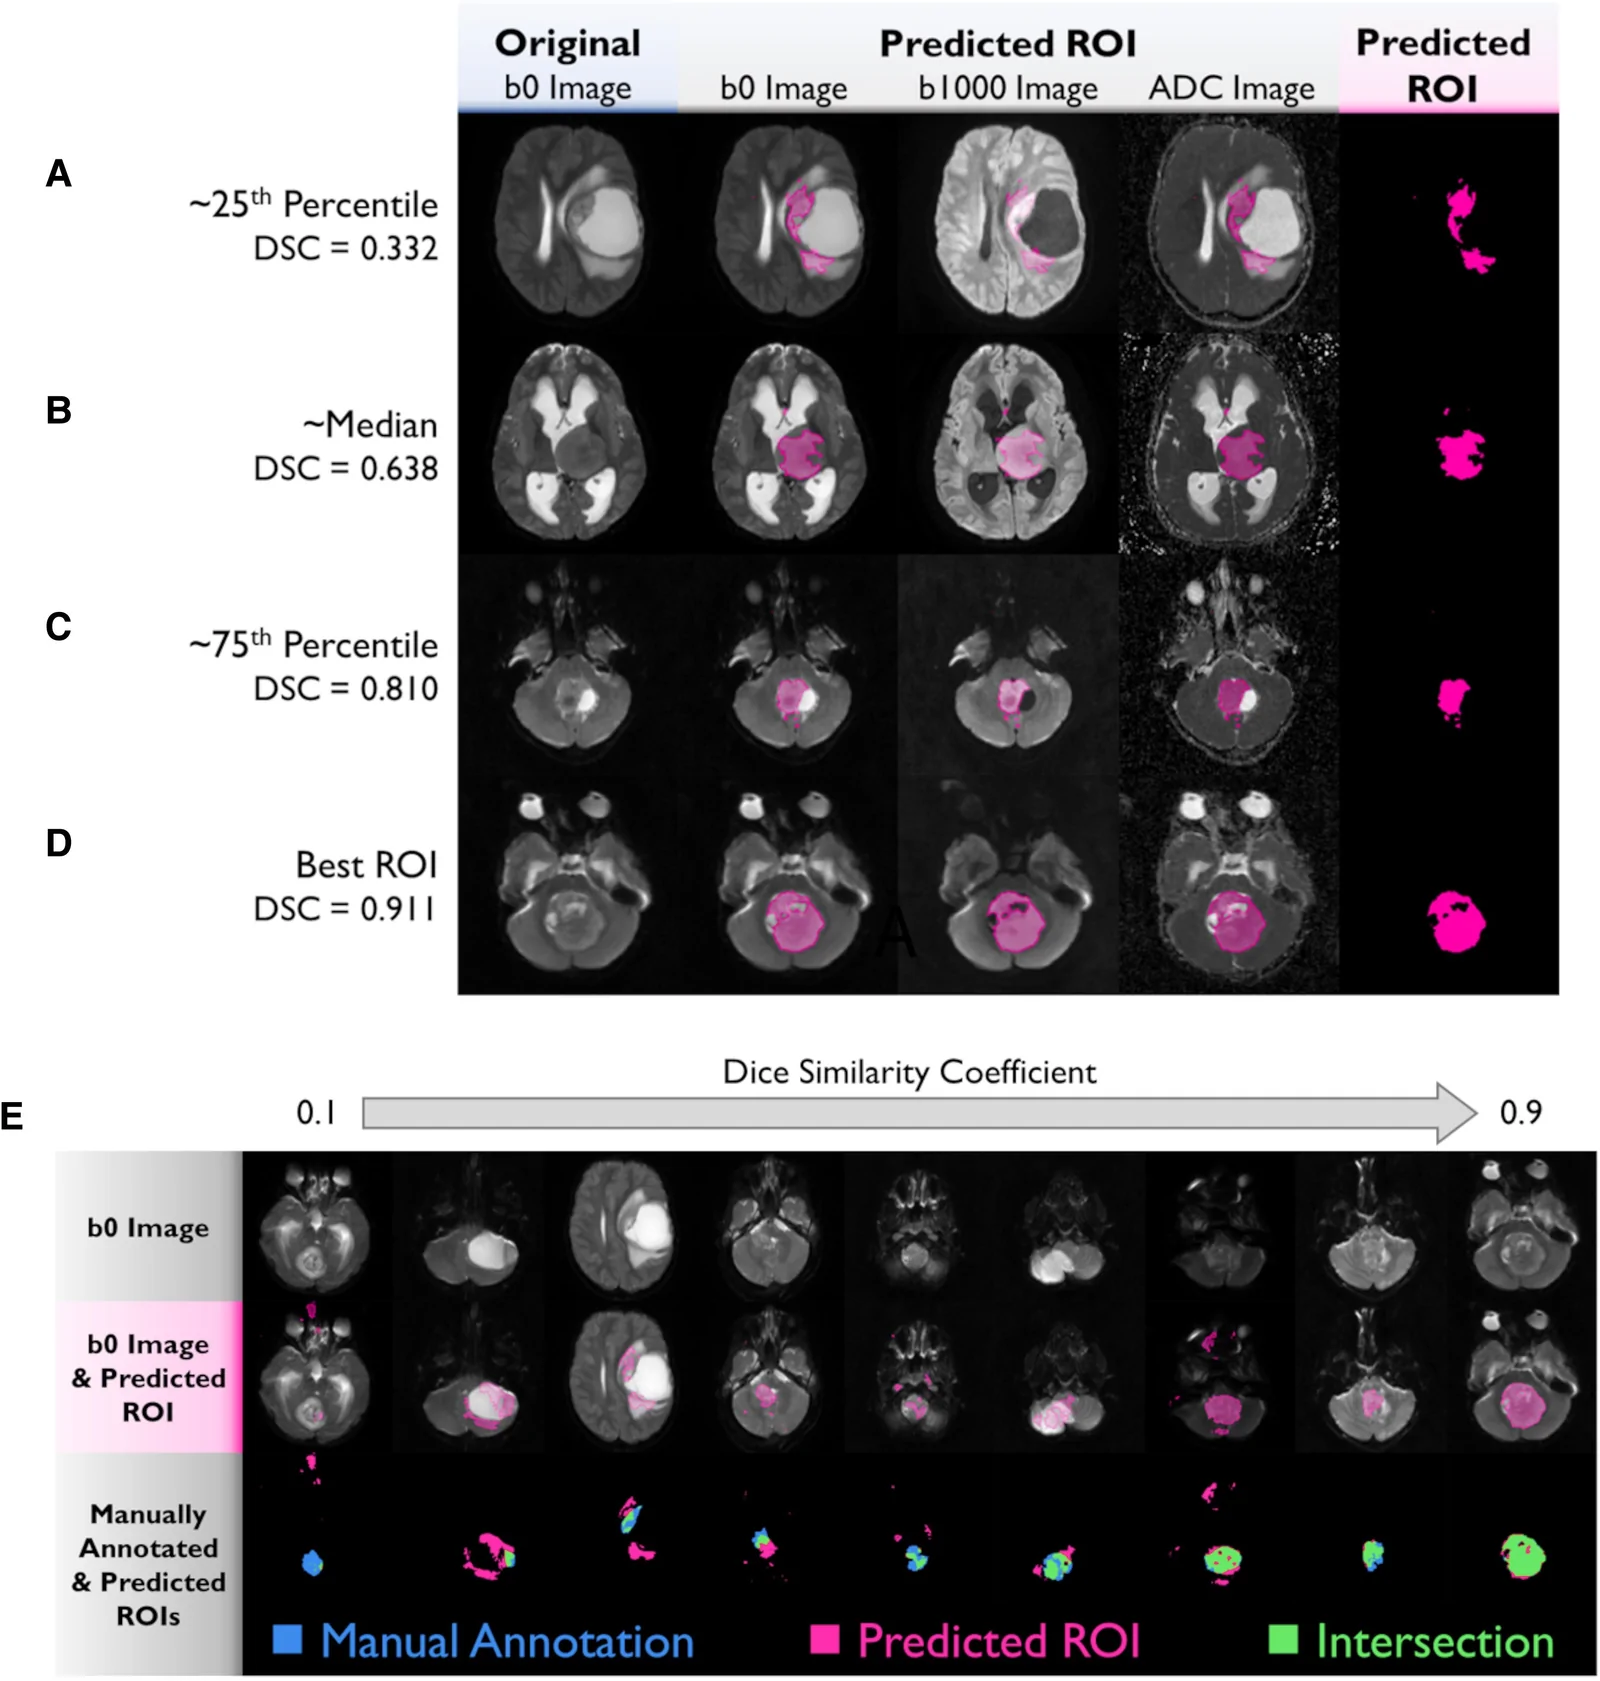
Example automatic segmentations of tumours. (A–D) Example predictions of increasing quality (from top row to bottom row), indicating performance on test fold, using the best-performing model (union-mask ensembled model). Rows represent individual patients selected based on their respective test-fold DSC, specifically selecting cases at given percentiles across the cohort: (A) 25th percentile (pilocytic astrocytoma patient); (B) 50th percentile, aka median (pilocytic astrocytoma patient); (C) 75th percentile (glioblastoma patient); and (D) best-performing case (medulloblastoma patient). Columns are (i) the b0 input image, (ii–iv) the predicted segmentation or ‘ROI’ overlaid upon the b0, b1000 and ADC input images (respectively) and (v) the predicted ROI. (E) To visually demonstrate the relationship between predicted segmentation and Dice score, a further selection of cases is displayed here. Cases were selected based on patient-level DSC; a case was sampled from each decile (from DSC = 0.1–0.9 in steps of 0.1) with performance closest to the desired DSC. The MRI slice visualized was selected based upon slice-level DSC, closest to the specific DSC decile. Cases have an increasing DSC from left to right. The top row shows the b0 image, the second row includes the predicted ROI overlaid on the b0 and the final row shows the intersection between the manual annotation and predicted ROI. This demonstrates the relative success of segmentations, even at mid-range deciles.
